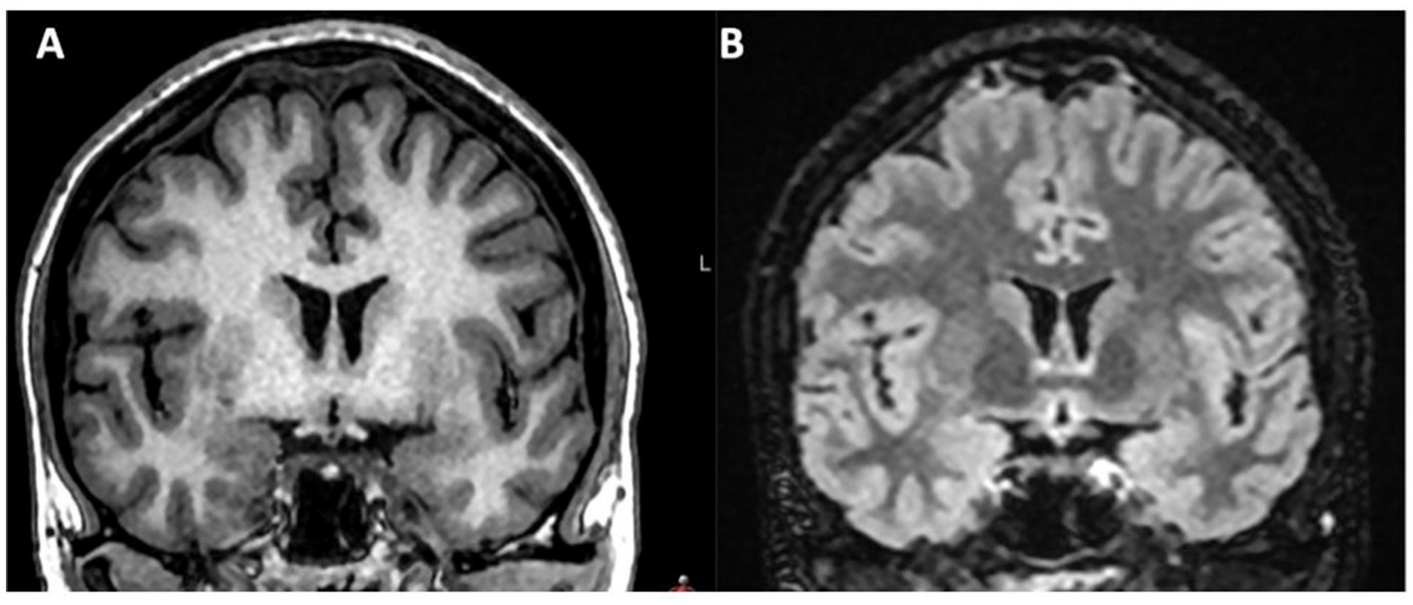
(A, B) Patient not presenting with IVLT after delivery with EDA, but signs of intracranial hypotension, with dilatation of parasagittal venous lakes. Coronal T1 MRI image and coronal T2 FLAIR image.
